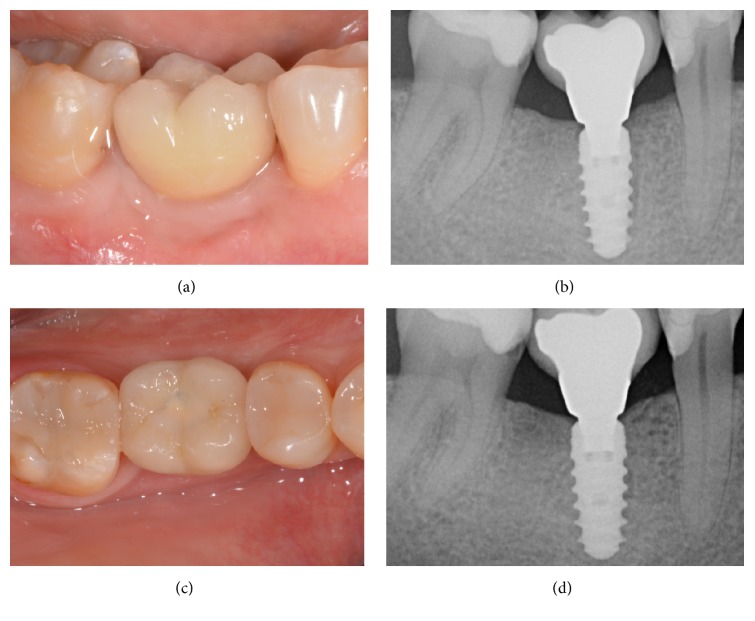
Immediate implant placement of a mandibular first molar (#46). Final metal-ceramic crown at delivery, lateral view (a); periapical radiograph of the implant at the delivery of the final crown (b); final metal-ceramic crown at delivery, occlusal view (c); periapical radiograph 2 years after implant insertion (d).
